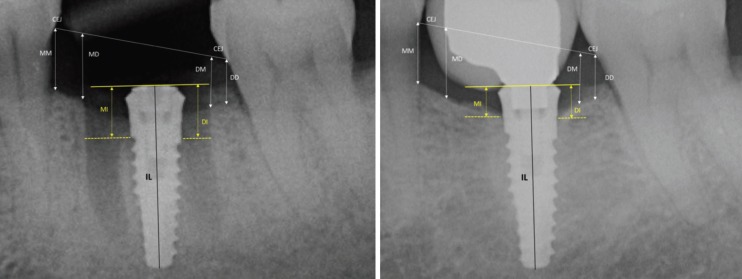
Example of the radiographic examination at the implant and membrane placement (T0) and at the end of the 3 years follow-up post-loading (T3). MM, MD = bone height at the mesial and distal aspects of the residual mesial extraction socket bone peak; DM and DD bone height at the mesial and distal aspects of the residual distal extraction socket bone peak, measured in mm from the line linking the CEJs of the adjacent teeth. MI and DI= peri-implant marginal bone mesial and distal levels.
